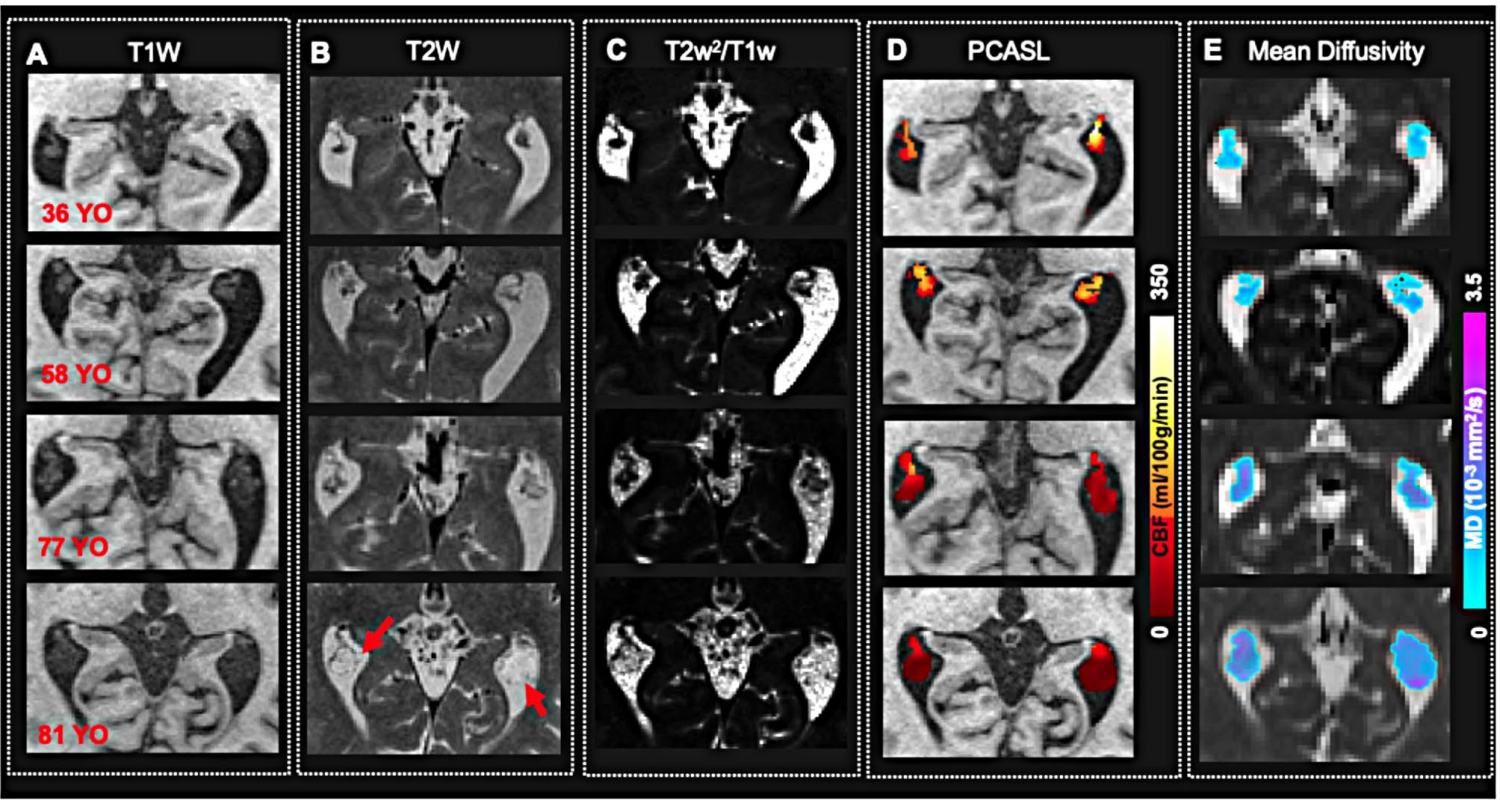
Representative images of ChP from individuals with different ages. (A) On T1w MRI, ChP volume increased with aging. (B) On T2 weighted MRI, the hypo-intensive flow-void vessels become sparser with cyst formation (red arrows) in older subjects. (C) By combining T1 and T2w data (T2w^2^/T1w), the contrast is enhanced, and the cyst delineation becomes easier. (D) CBF within the ChP on pCASL decreases with aging. (E) ChP MD values increase with aging
